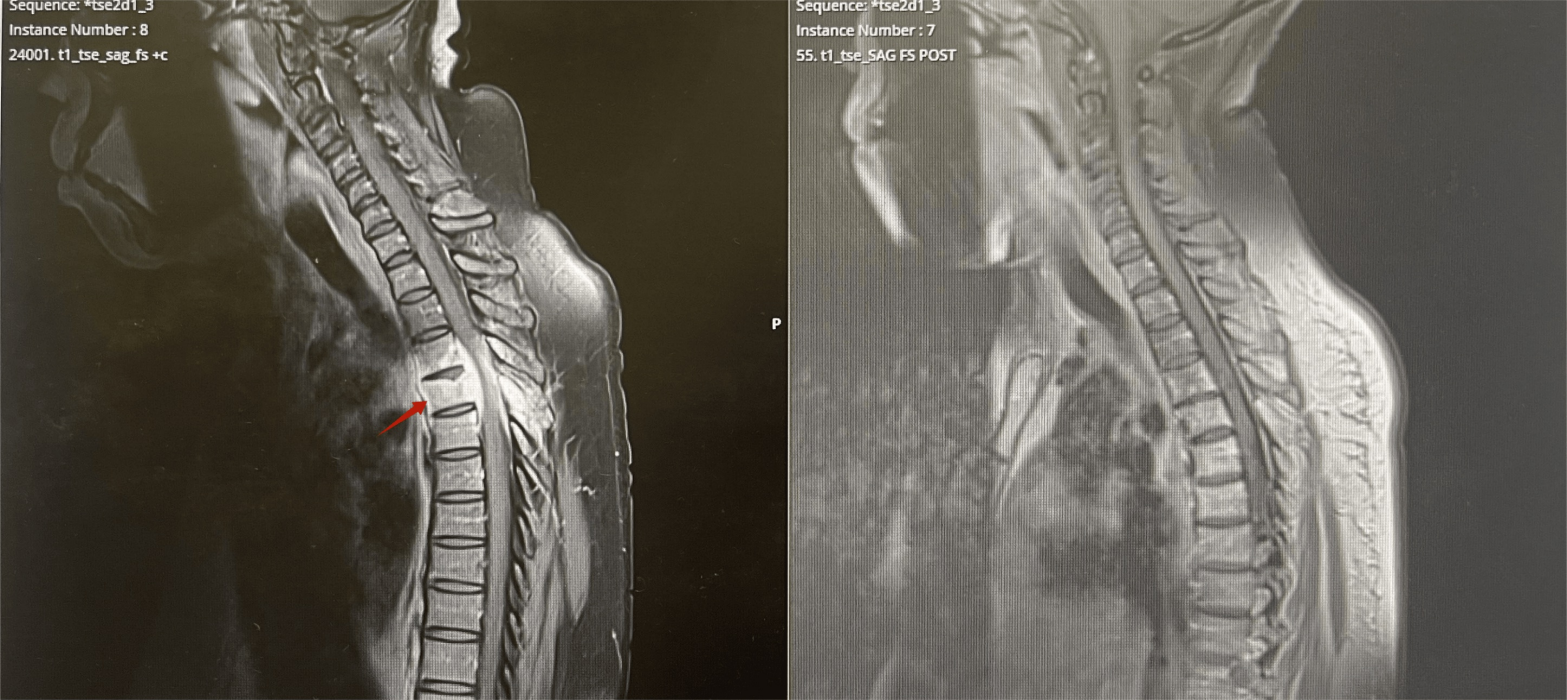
MRI of the spine before (left) and two months after (right) initiation of anti-tubercular therapy. Post-treatment image shows marked resolution of paravertebral enhancement and improved spinal cord decompression compared to pre-treatment MRI (arrow).
